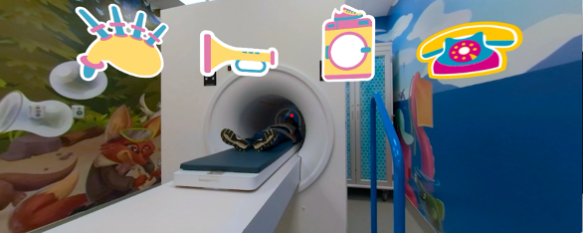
A screenshot of narrative elements used to describe the magnetic resonance imaging sounds.
